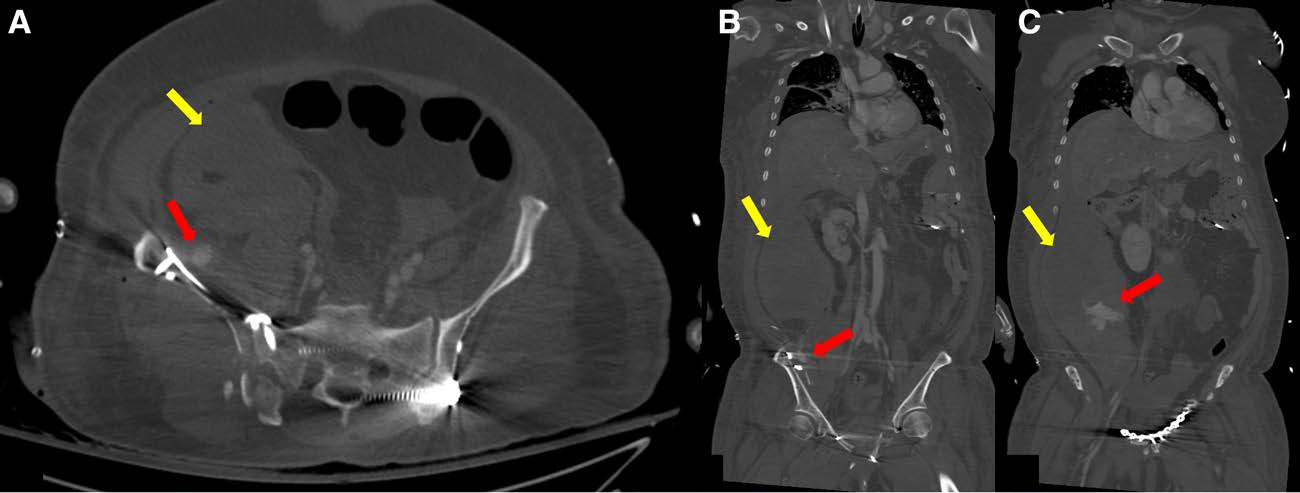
(A) Coronal and (B, C) axial views of computed tomography demonstrating a massive hematoma (yellow arrows) with extravasation of contrast media (red arrows).
